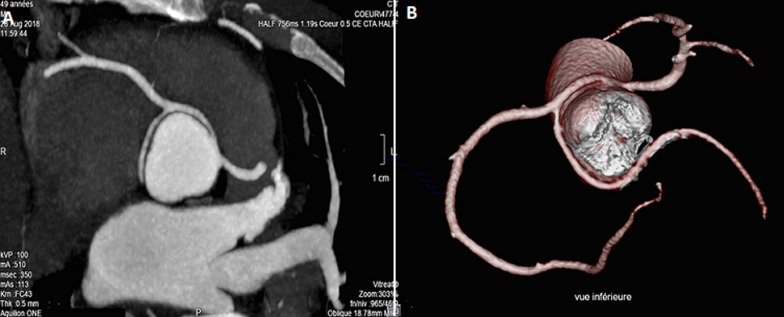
CT coronary artery, A) transverse view showing anomalous origin of right coronary artery with interarterial course (the birth of a common trunk from the left anterior sinus passing between the aorta and the pulmonary artery and giving right coronary artery and circumflex artery); B) inferior view of a three-dimensional reconstruction demonstrating arterial paths
